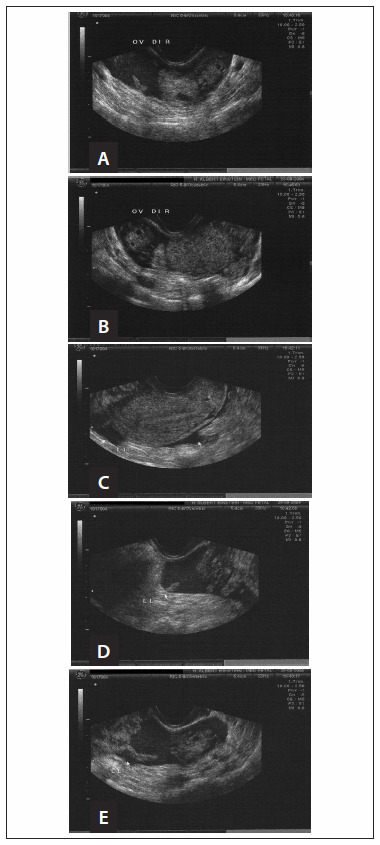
Transvaginal ultrasound on the ectopic pregnancy. Left (A and B): Right ovarian and paraovarian regions. Examination performed due to a late menstrual period, vaginal bleeding and abdominal pain. Image suggestive of tubal pregnancy. Right (C, D e E): Image suggestive of free-flowing fluid (identified as LL) in the pouch of Douglas, as confirmed by the large quantity of blood observed intraoperatively.
